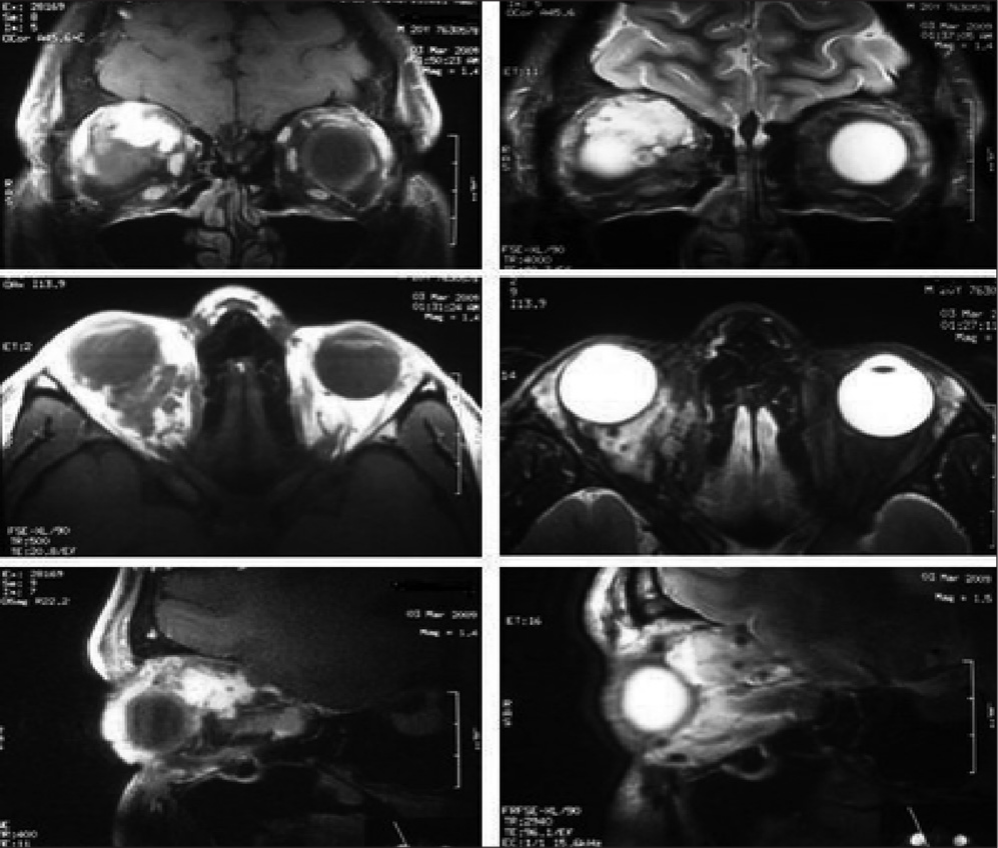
Coronal (T1-w contrast, T2 w/out contrast), axial (T1 and T2), and sagittal (T1 and T2) slices of MRI
